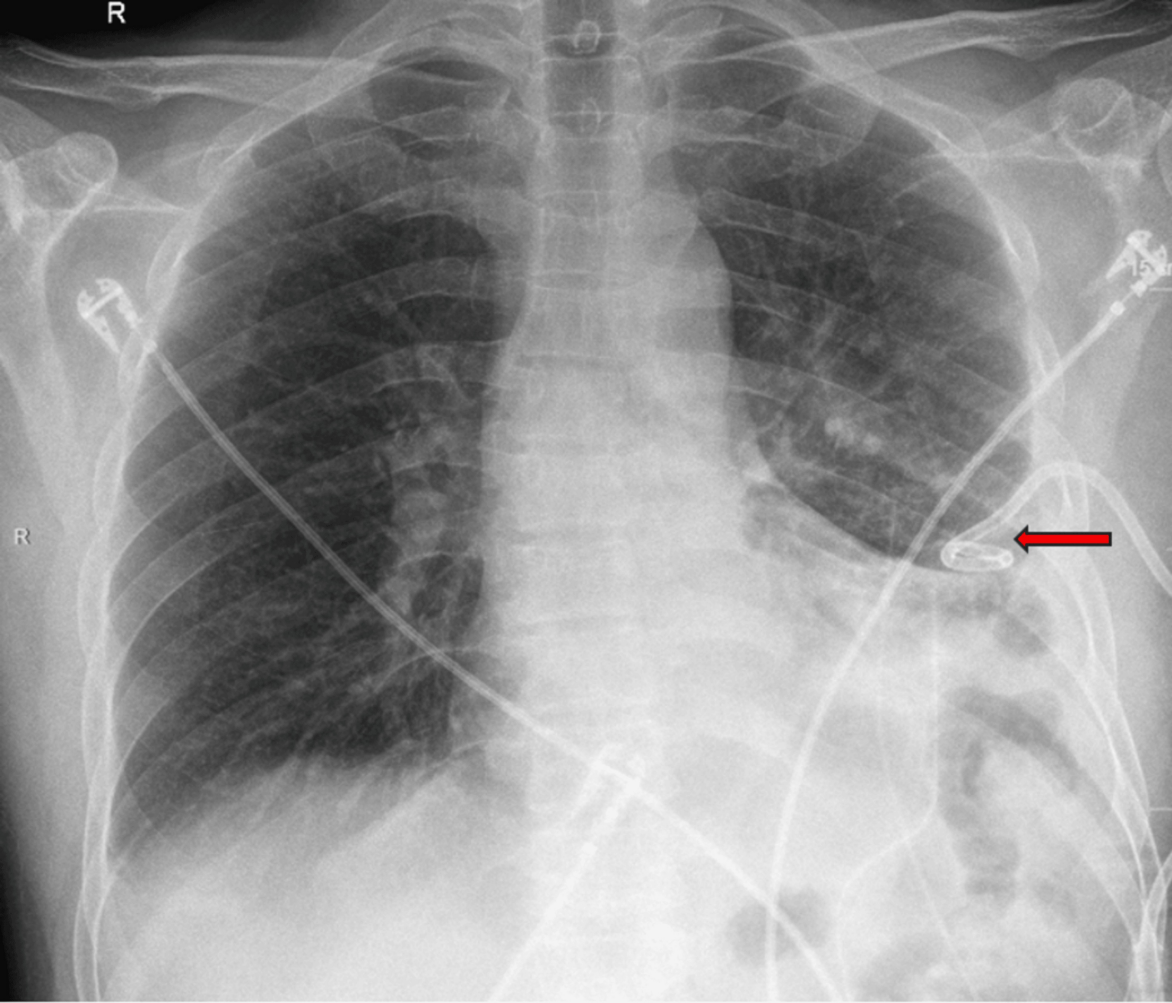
X-ray of the chest Red arrow indicating the left-sided infiltrate, pleural effusion, and chest tube in place.
